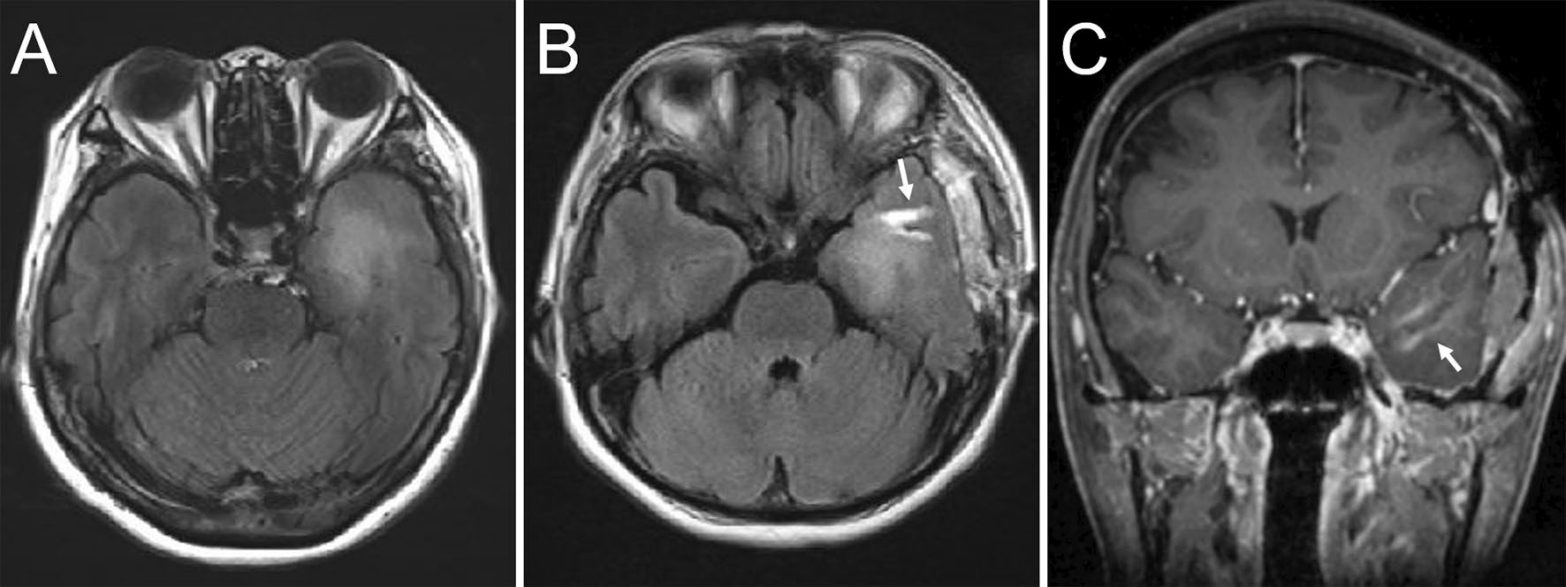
Preoperative magnetic resonance imaging (MRI) scans showing fluid-attenuated inversion recovery hyperintensity lesion at the left temporal lobe (A). Postoperative MRI scans, following boring biopsy twice revealed that column cavity from the surface to the core of the lesion is present without hematoma and surrounding brain edema (B,C).
